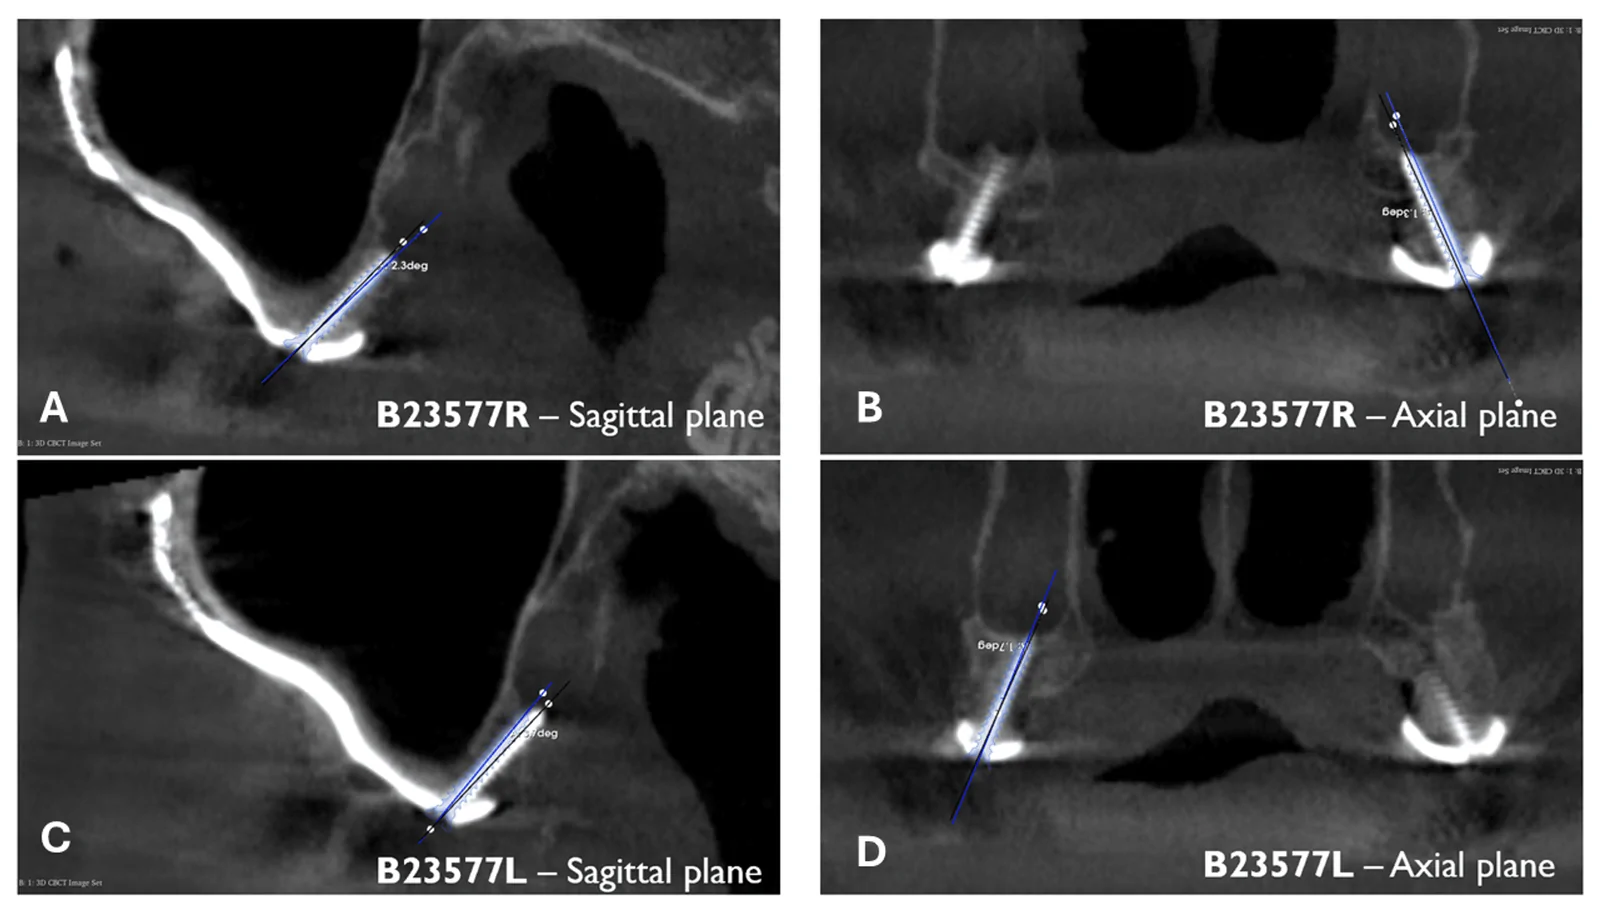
Comparison between virtual planning and postoperative pterygoid screw positions. A: sagittal CBCT view of the right side (B23577R); B: axial CBCT view of the right side (B23577R); C: sagittal CBCT view of the left side (B23577L); D: axial CBCT view of the left side (B23577L). The virtual planned trajectories are shown in blue, and the postoperative pterygoid screw positions are shown in white. The observed angular deviations demonstrate the accuracy of the prosthetic-guided placement protocol.
